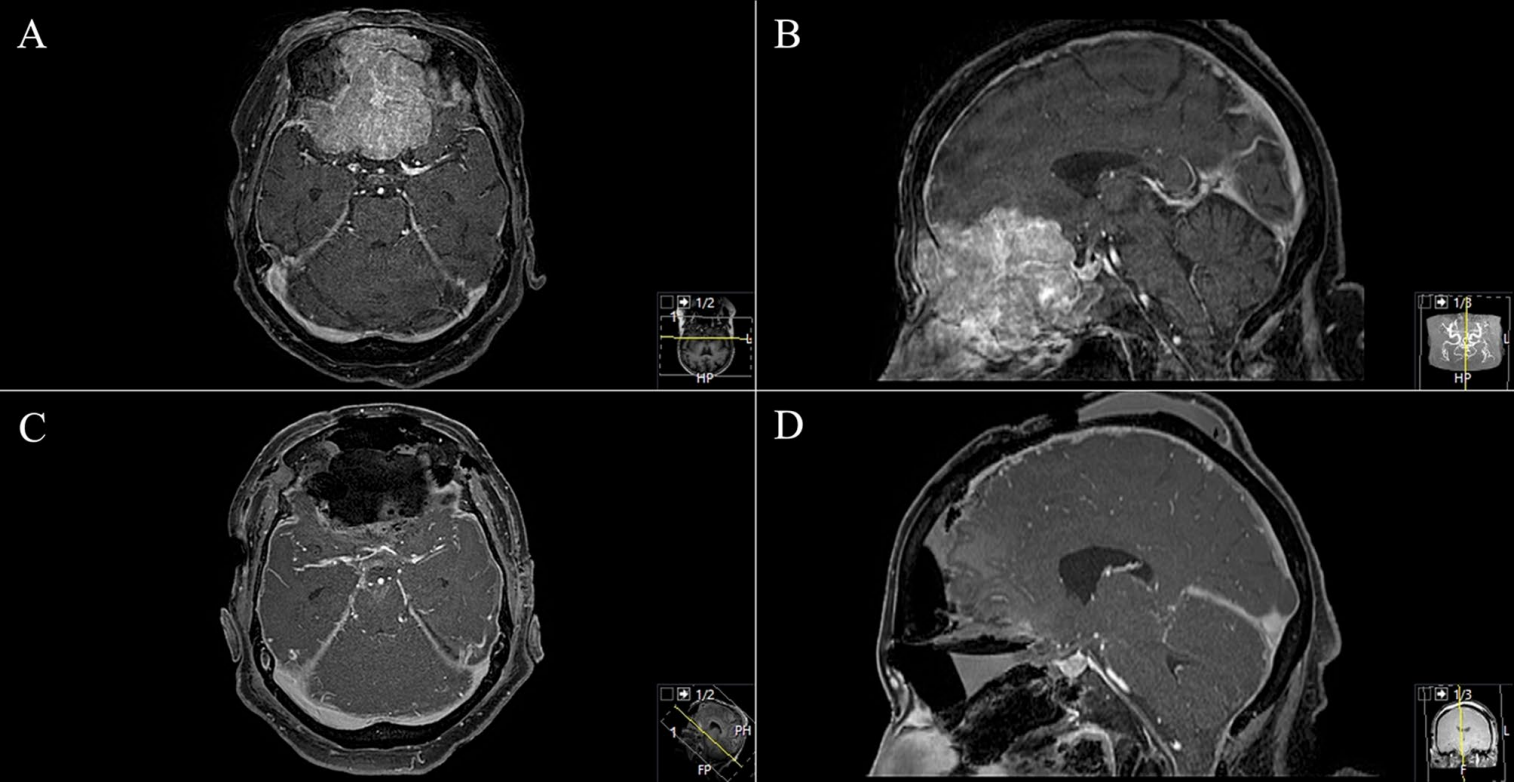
Exemplary case 1: a 74-year-old female patient admitted after fall at home and back pain. Cranial routine CT scan displayed a huge transbasal bone infiltrating and destroying a tumor with sinonasal affection. (A,B) Preoperative T1-weighted gadolinium-enhanced MR imaging showing sinonasal and orbit-infiltrating tumor with subtotal anterior skull base destruction. (C,D) Postoperative MR control showing successful resection. Reconstruction was performed with split-bone graft and galea-periosteum flap. Pathologic findings revealed adenoid cystic carcinoma. The patient recovered well from the procedure; she underwent VP-shunt implantation in further course.
